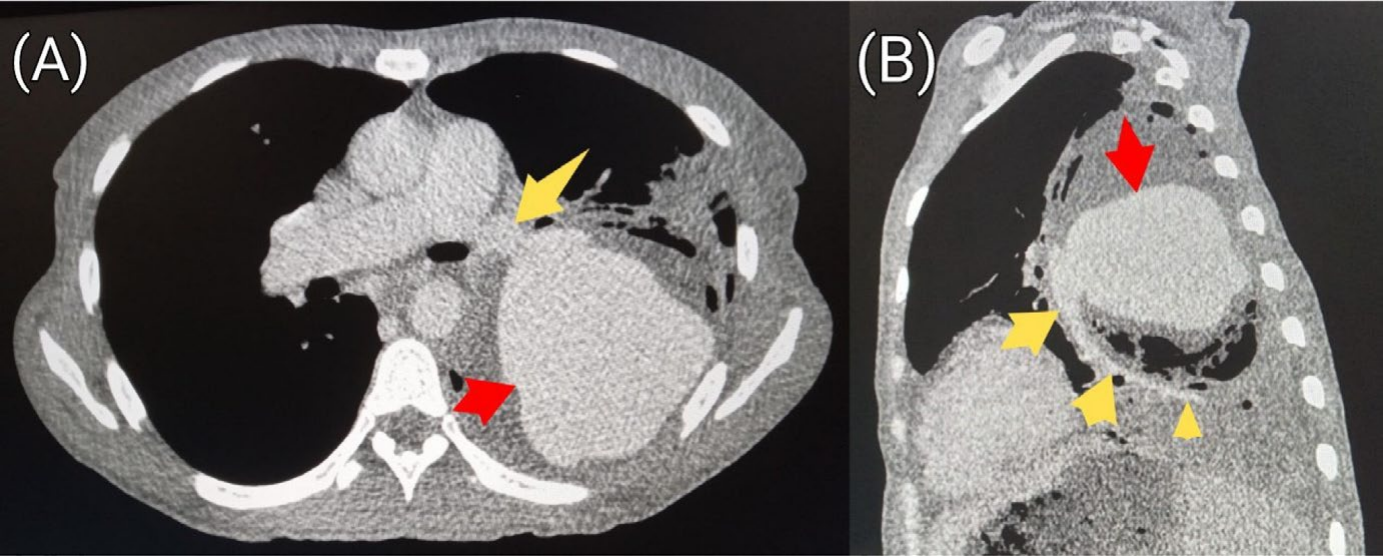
Post‐contrast axial (A) and sagittal (B) chest CT images (soft tissue window) showing an aneurysmal dilatation (red arrow) arising from the interlobar branch of the left pulmonary artery (yellow arrow).
